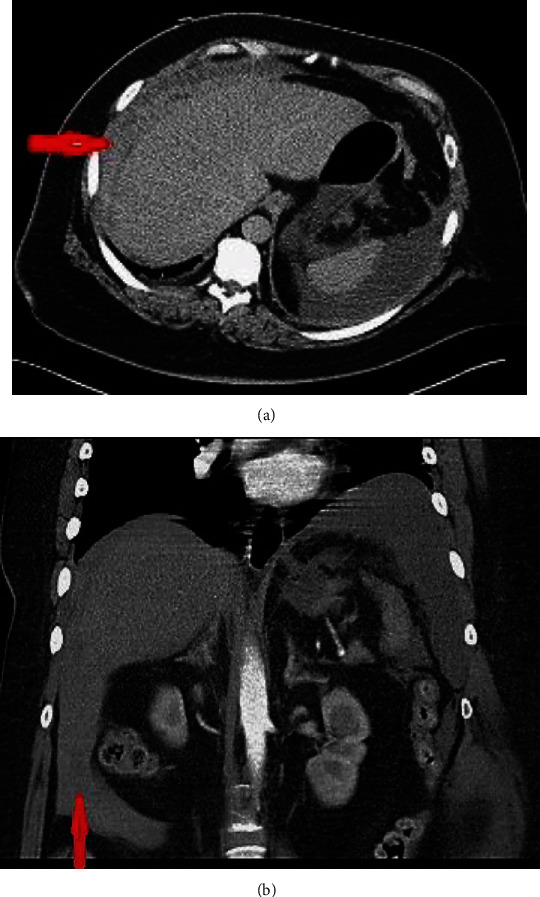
(a) Axial postcontrast CT image demonstrating acute subcapsular haematoma surrounding the lateral surface of the right lobe of liver (red arrow). (b) Coronal postcontrast CT image demonstrating ruptured subcapsular haematoma with fresh blood extending to the right paracolic gutter (vertical arrow points to a hyperdense material inferior to the liver).
